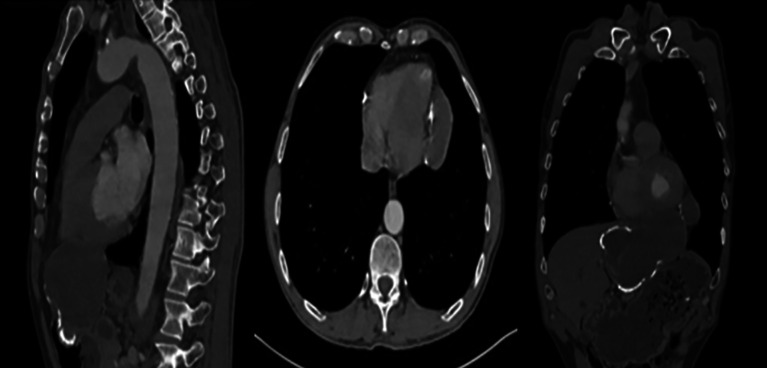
CT scan showing the hydatid liver cyst passing through Larrey’s hiatus.
